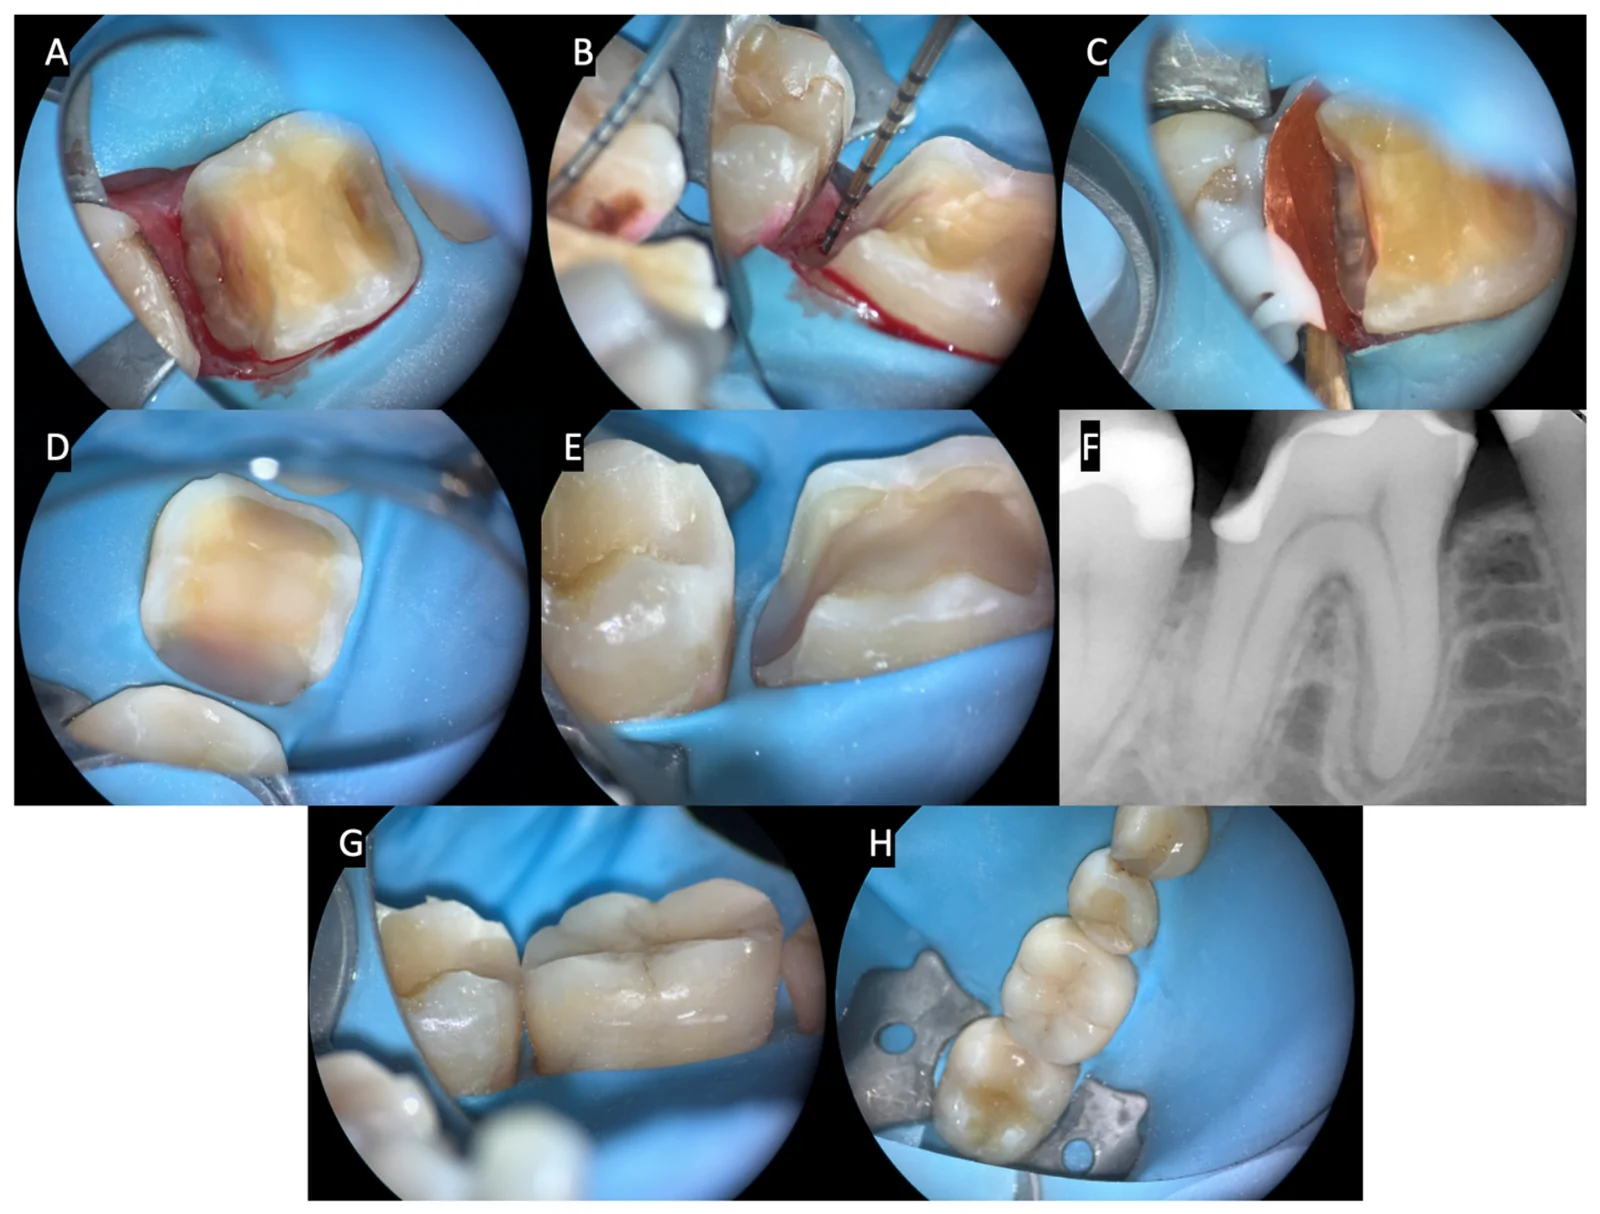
Zone 2 clinical scenario. (A) Initial occlusal view; (B) initial lateral view with evaluation of margin depth using a periodontal probe; (C) occlusal view after placement of the matrix; (D) occlusal view after completion of deep margin elevation; (E) lateral view after completion of deep margin elevation; (F) radiograph of the deep margin elevation; (G) lingual view of the final full-coverage crown; and (H) occlusal view of the final full-coverage crown.
